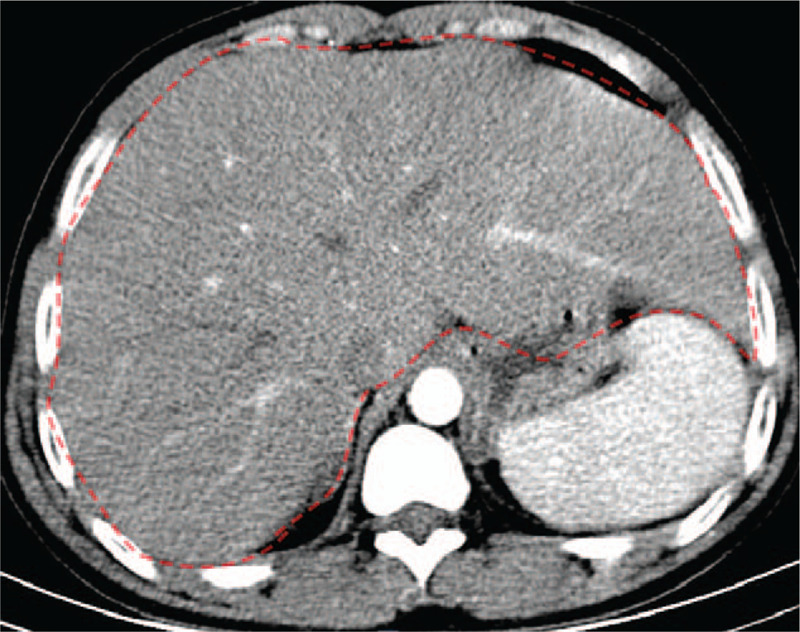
Abdominal contrast-enhanced computed tomography image displaying hepatomegaly (red area).
